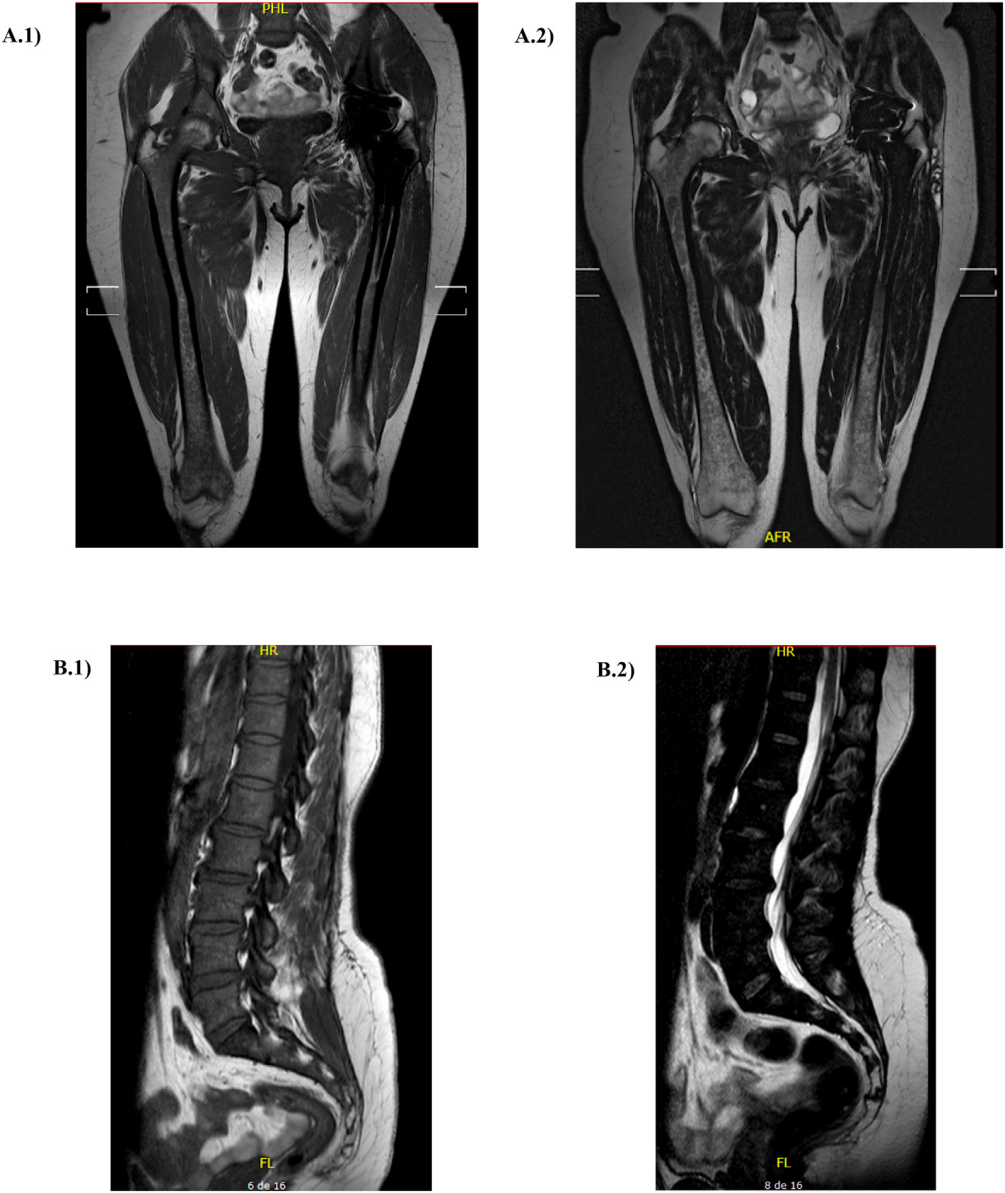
MRI images of patient 2 at baseline. A.1) Coronal T1-weighted image of femurs. A.2) Coronal T2-weighted image of femurs. B.1) Sagittal T1-weighted image of lumbar spine. B.2) Sagittal T2-weighted image of lumbar spine. Femur total score = 7 (T1 = 2, T2 = 2, Sites = 3); Lumbar spine total score = 7 (T1 = 2, T2 = 2, Pattern = 3).
